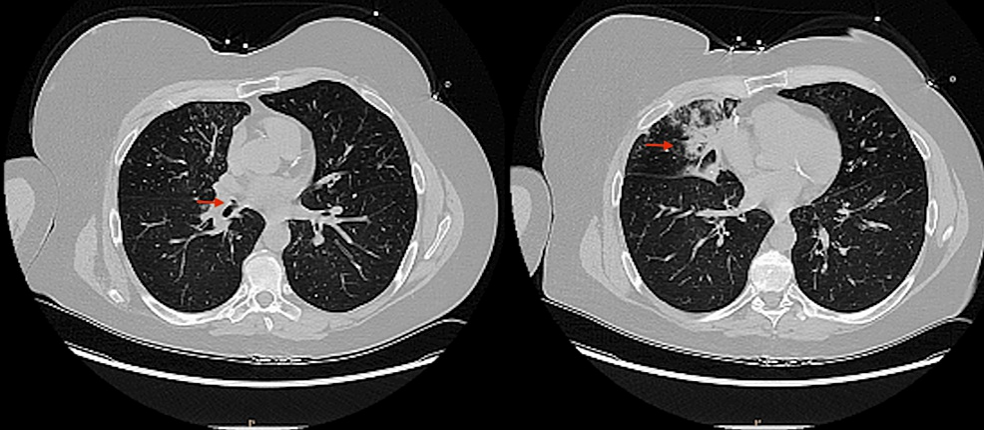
Chest CT images showing occlusion at the right middle lobe bronchus origin with consolidation/cavitation (red arrows)
